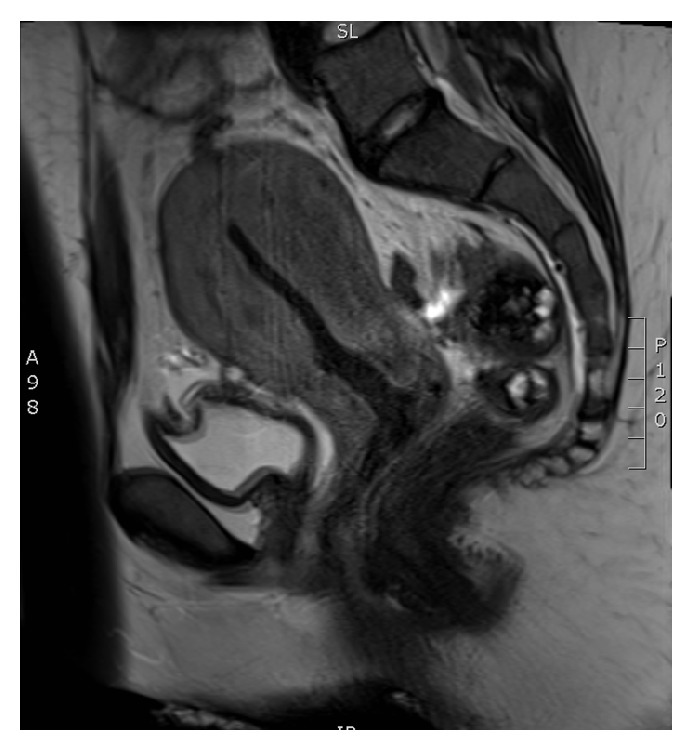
Pelvic MRI showing a large uterus with disappearance of its zonal anatomy, yet lacking any signs of an endometrial tumor.
